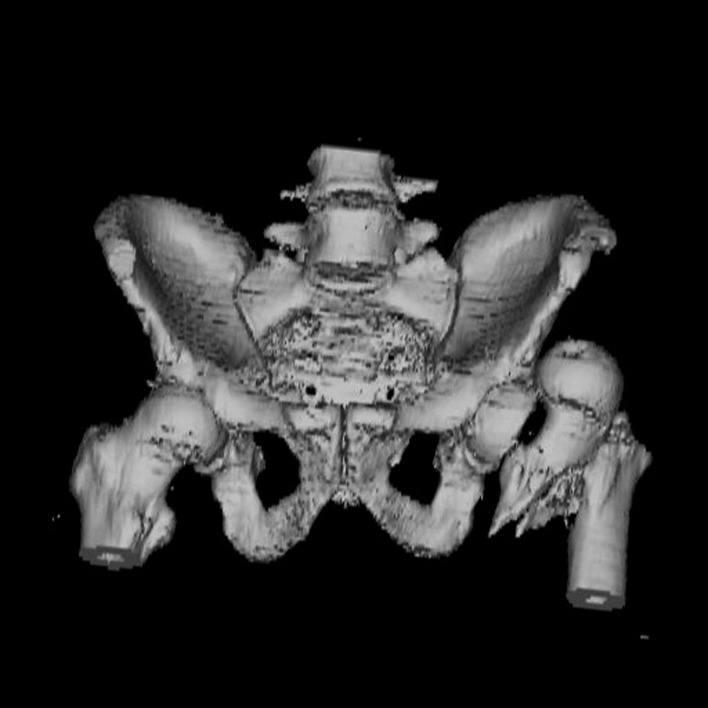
3D CT reconstruction of the pelvis showing anterior displacement of the left femoral head and intertrochanteric fracture. No other osteoarticular injuries were found
